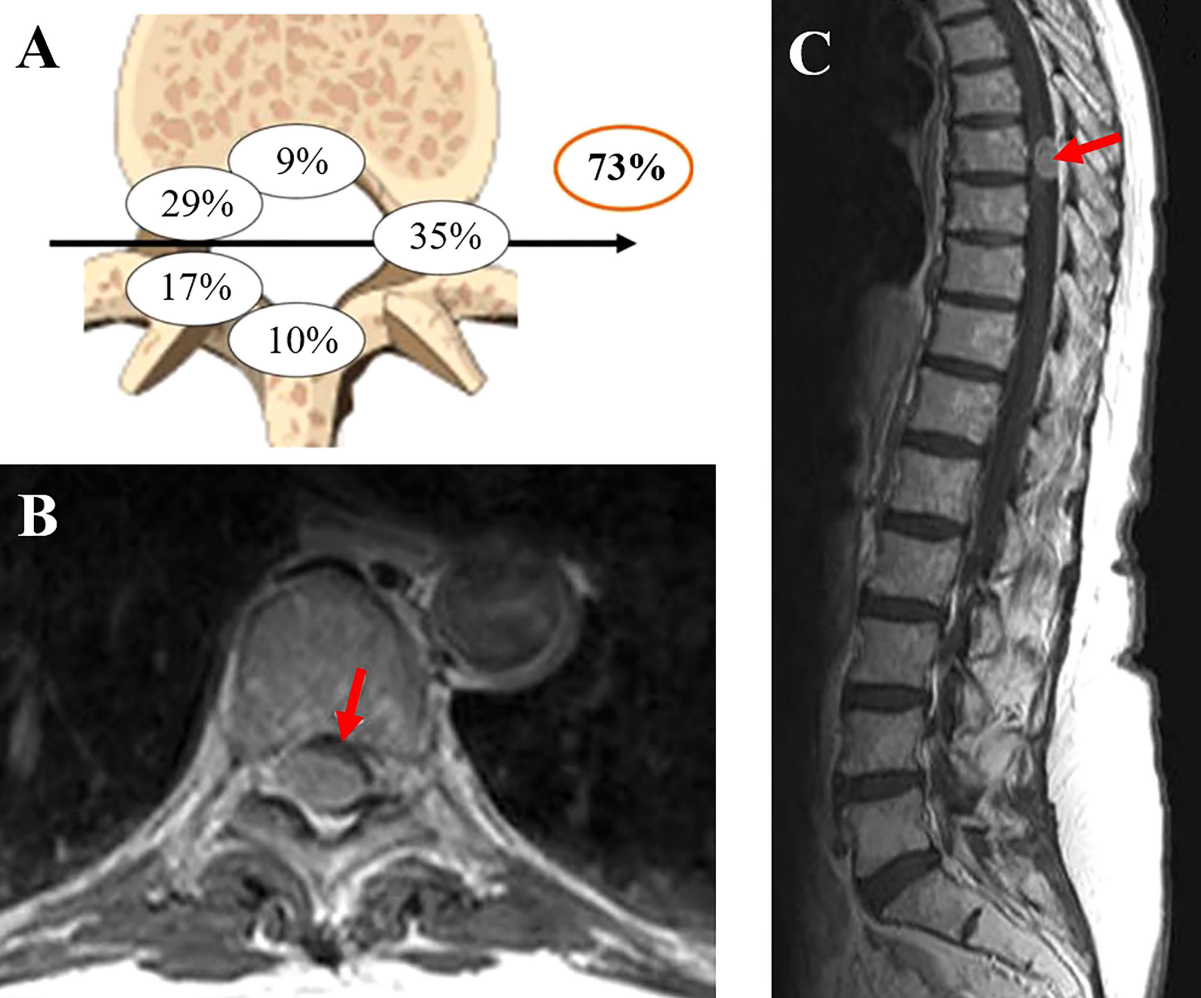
(A) Localization of the dural attachment (73% located ventrally to the dentate ligament). (B, C) Spinal meningioma aspects on MRI. The figure is adapted from reference 4 and reproduced with permission from SNCSC.
